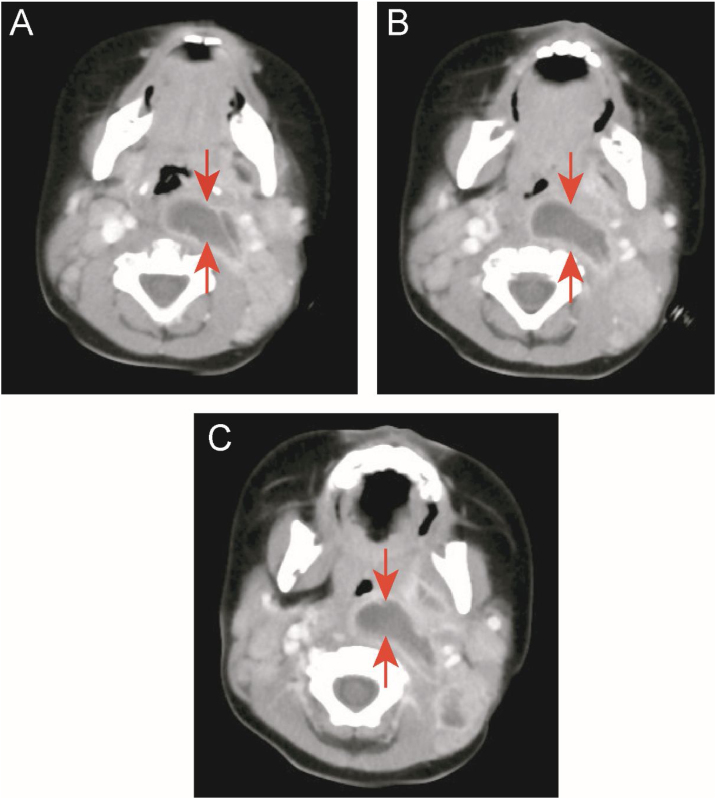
Case 1: a patient with KD and deep neck abscess who underwent incision and drainage surgery. (A, B, and C) Enhanced neck computed tomography showing a neck abscess.
